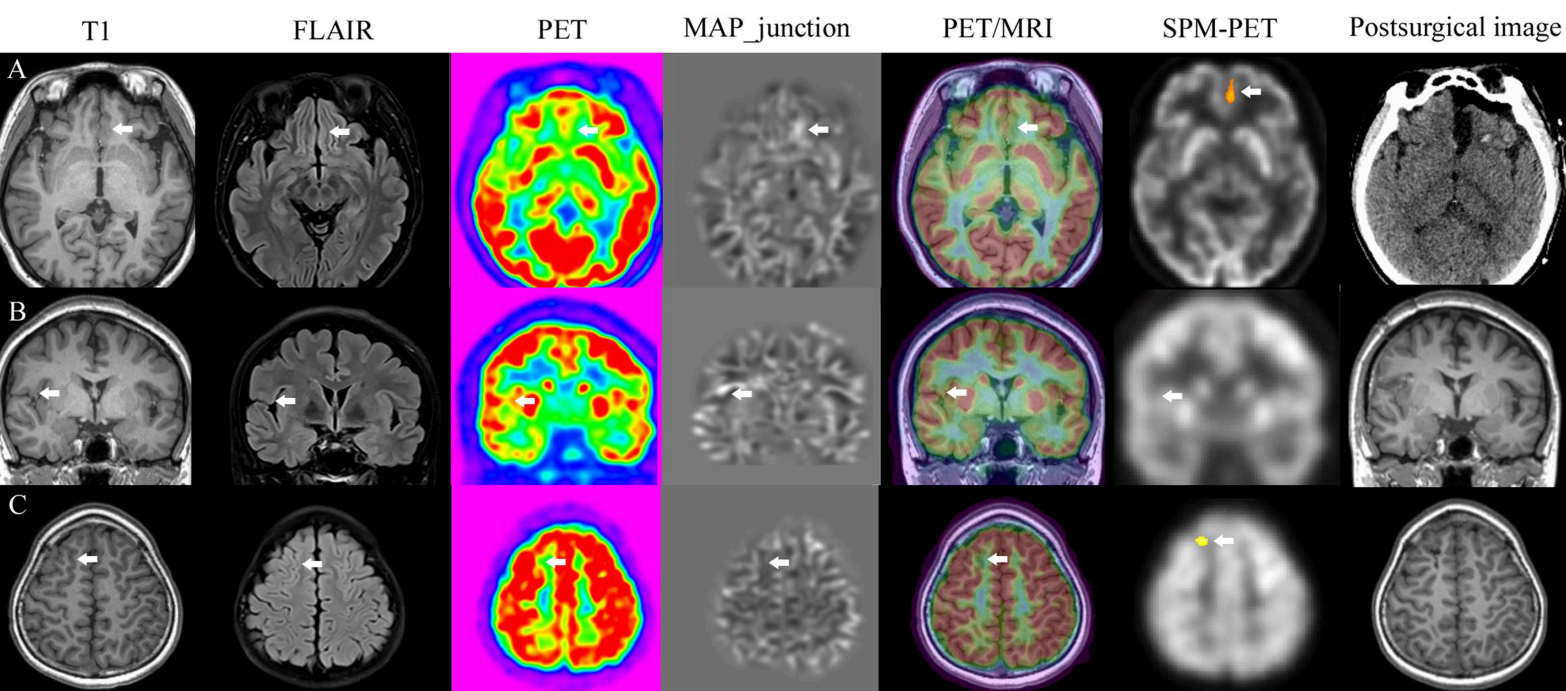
Image post-processing examples. Patients with FCD lesions detected by all methods (A), only MAP (B), PET/MRI, and SPM-PET (C).
